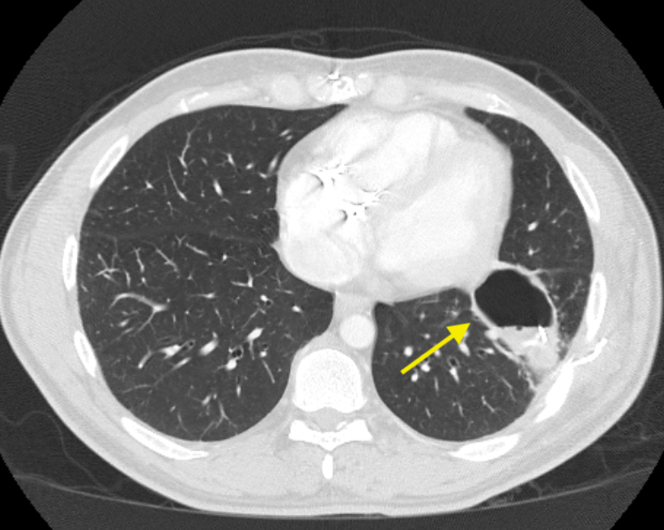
Chest CT Showing A Left Lower Lobe Cavitary Lesion With Retained Epicardial Leads (Arrow), Consistent With Pulmonary Abscess
